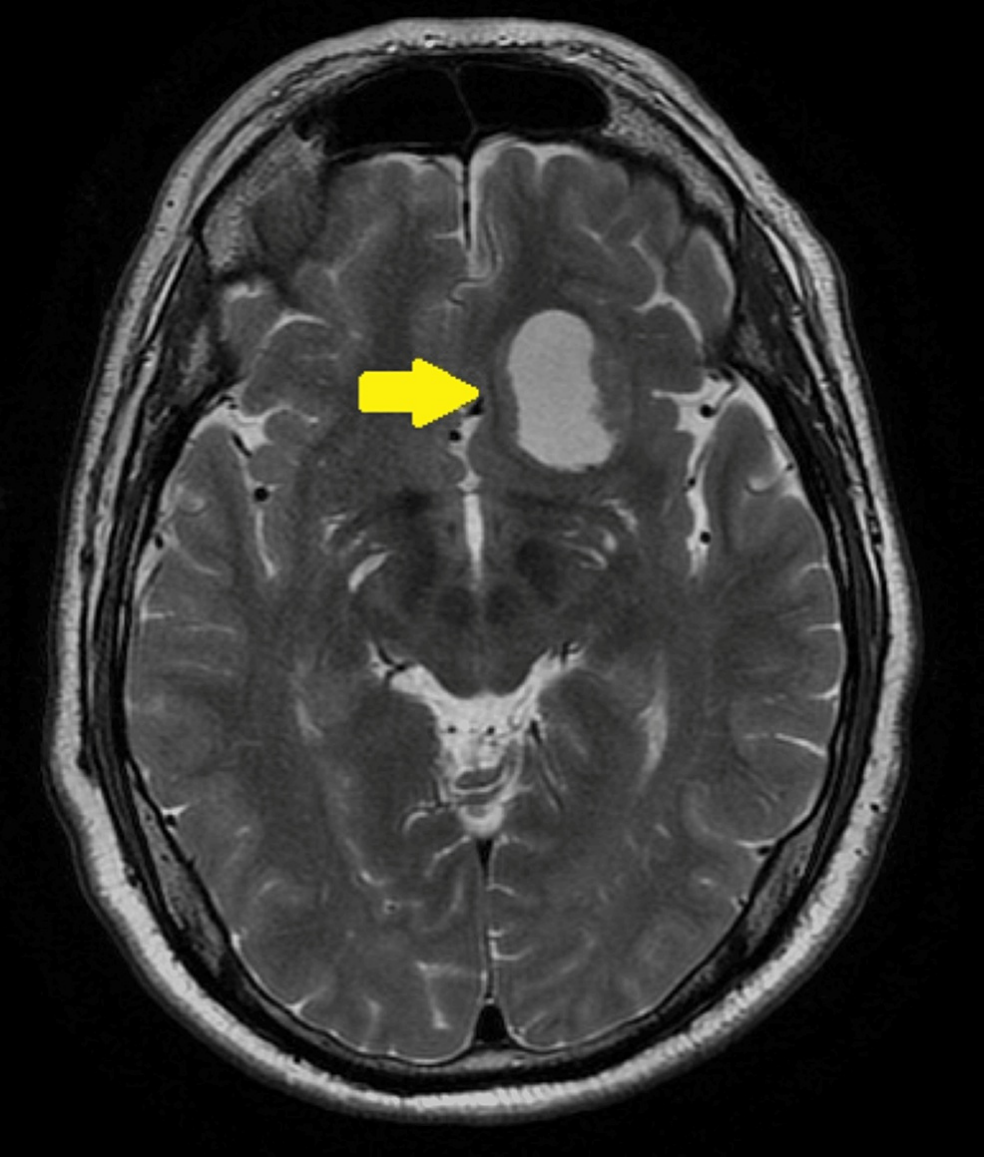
Pre-operative brain magnetic resonance imaging showing an endocranial expansile lesion measuring approximately 33 mm in largest diameter.
